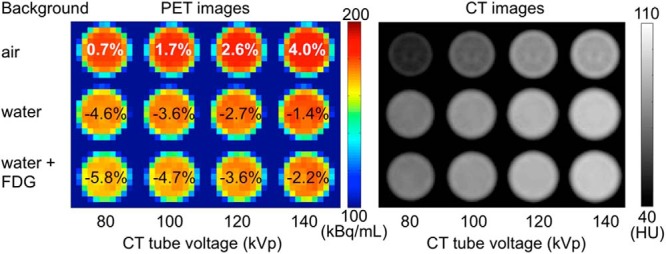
Changes in signal from PET images as background media and CT tube voltage are varied. The CT images on the right were used for attenuation-correction in the generation of the corresponding PET images on the left. Percentage values over the PET images are the biases measured for the central XCaliper region of interest (ROI) versus known activity concentration.
